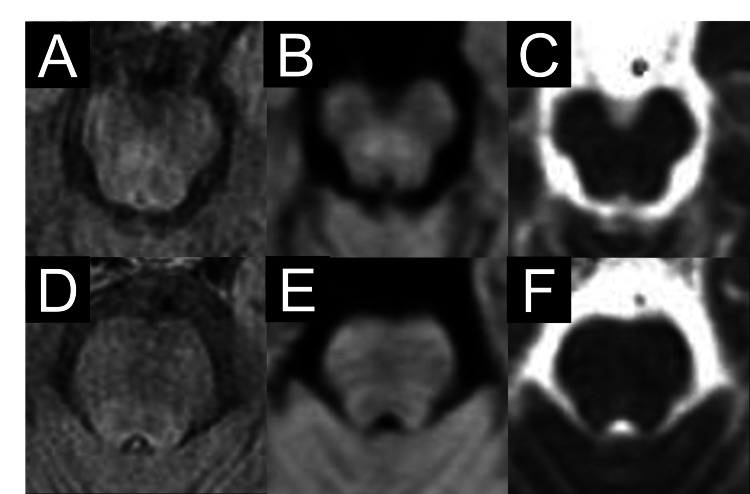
MRI findings on day 10 of admission Hyperintensity in the midbrain tegmentum, sparing the cerebral crus, resolved on FLAIR imaging (A), DWI (B), and the ADC map (C). Similarly, hyperintensity in the pontine tegmentum resolved on FLAIR (D), with corresponding resolution of hyperintensity on DWI (E) and low values on the ADC map (F). ADC: Apparent diffusion coefficient; DWI: Diffusion-weighted imaging; FLAIR: Fluid-attenuated inversion recovery
